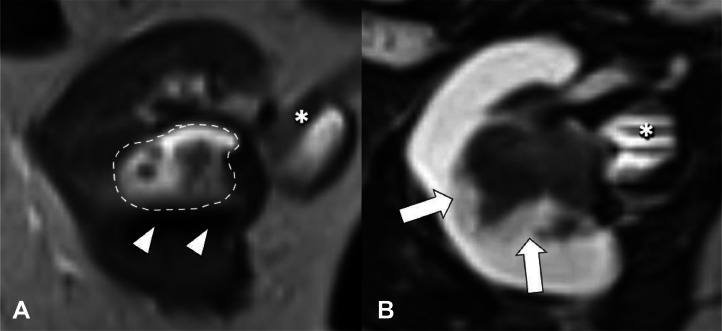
MRI of the right kidney. (A) T2 axial MRI shows hyperintense diverticulum (dotted lines) with hypointense layering calcifications/calculi (white arrowheads) (B) Contrast enhanced axial T1 image show enhancing nodules (white arrows) and contrast excretion into the renal pelvis (white *).
